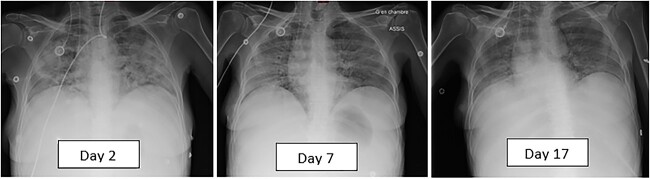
Successive chest X-rays on the second, seventh, and seventeenth day of hospitalization, showing regression of diffuse opacities with corticosteroid therapy.
